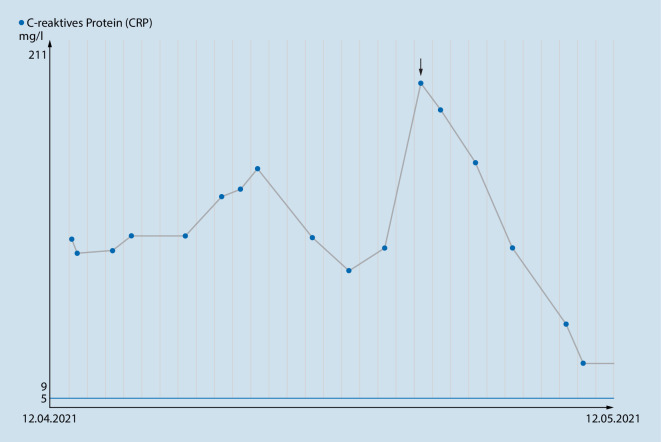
In einer ambulanten Kontrolle in unserer Klinik nach etwa drei Monaten zeigte sich der Patient beschwerdefrei. Die Therapie mit Prednisolon war wie geplant vier Wochen zuvor beendet worden. Die Sonographie der Schilddrüse ergab eine normale Organgröße (Gesamtvolumen 11 ml) und ein homogenes echonormales Binnenreflexmuster ohne Nachweis von fokalen Läsionen (Abb. 4). Im Labor zeigten sich eine euthyreote Stoffwechsellage sowie ein normwertiges CRP (Tab. 1). Somit lag eine vollständige Ausheilung der subakuten Thyreoiditis vor.
